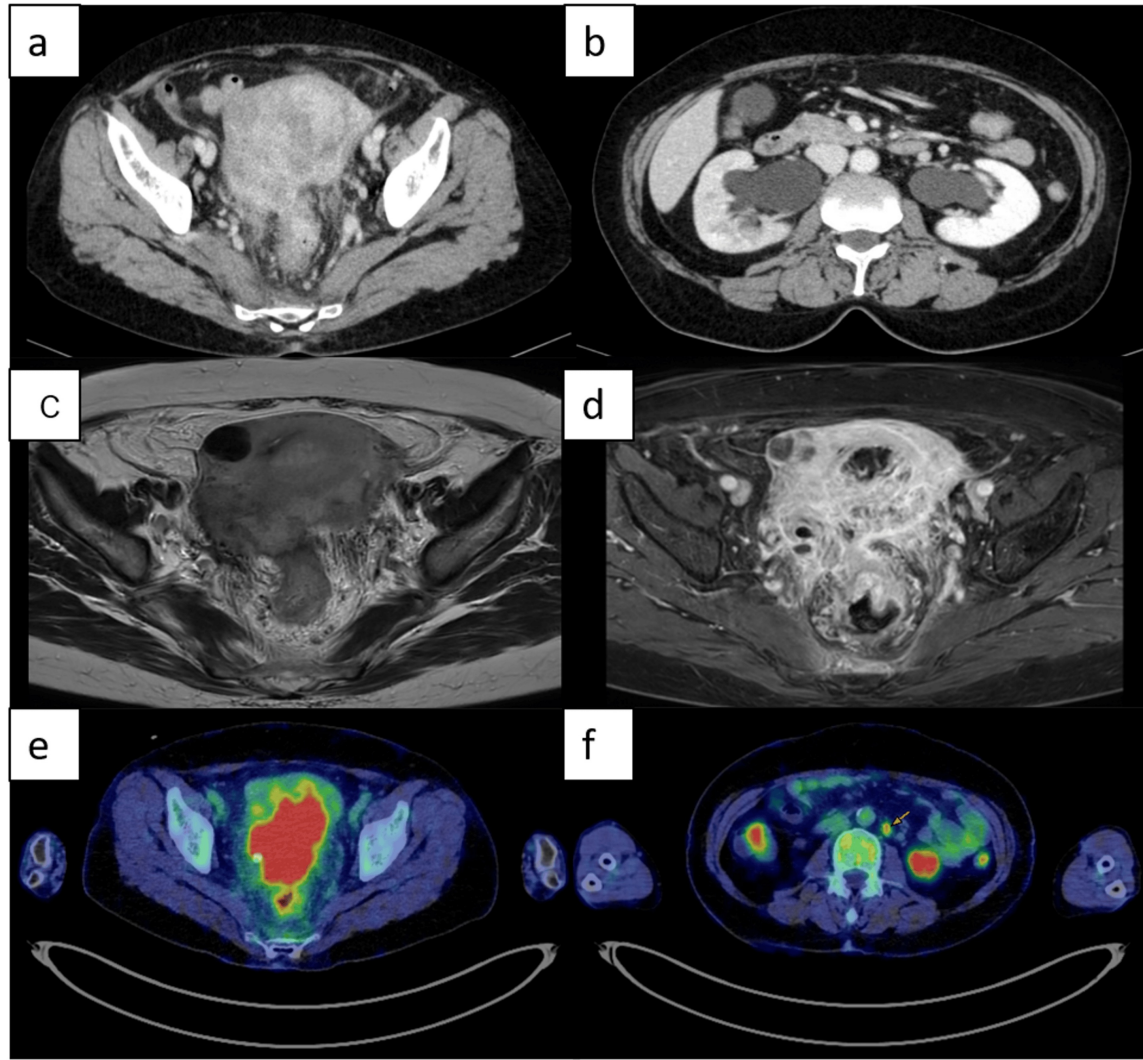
Imaging findings of the patient CT: computed tomography; PET-CT: positron emission tomography/computed tomography; MRI: magnetic resonance imaging; FDG: fluorodeoxyglucose (a, b) Contrast-enhanced CT showing an enlarged uterus and bilateral hydronephrosis and hydroureter. (c) T2-weighted MRI showing a heterogeneous signal intensity from the cervix to the body of the enlarged uterus. (d) Contrast-enhanced MRI showing heterogeneous contrast enhancement. (e, f) PET-CT showing intense FDG uptake in the mass extending from the cervix to the uterine body and in the paraaortic lymph node (yellow arrow)
